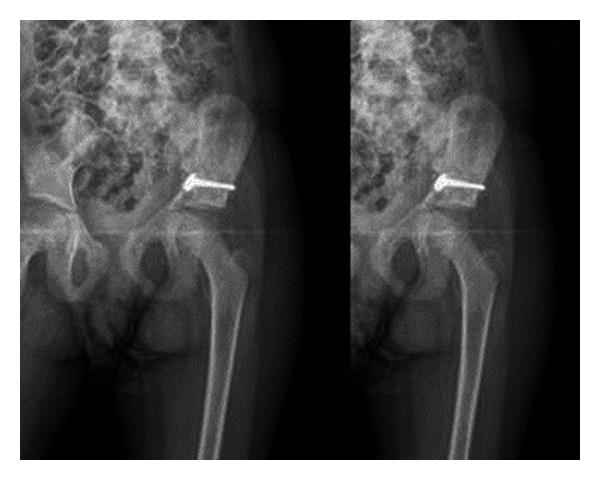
Twelve-month postoperative pelvis AP X-ray showing no cartilage damage.
